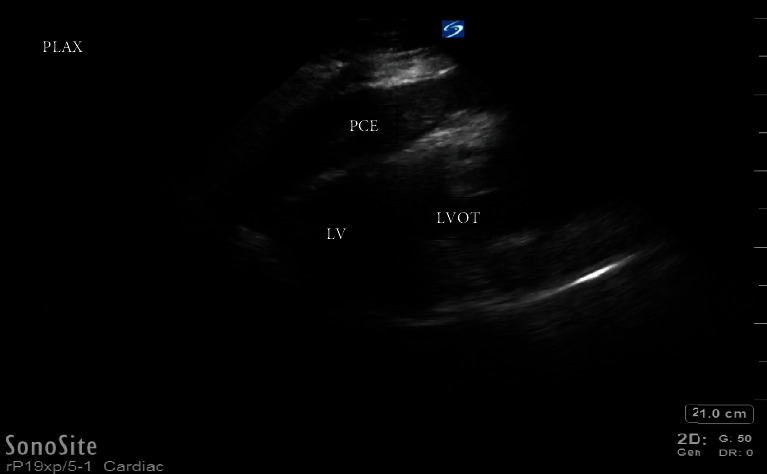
Parasternal long axis view of the heart displaying a pericardial effusion with dilated aortic root, concerning for aortic root dissection. LV: left ventricle; LVOT: left ventricular outflow tract; PCE: pericardial effusion.
